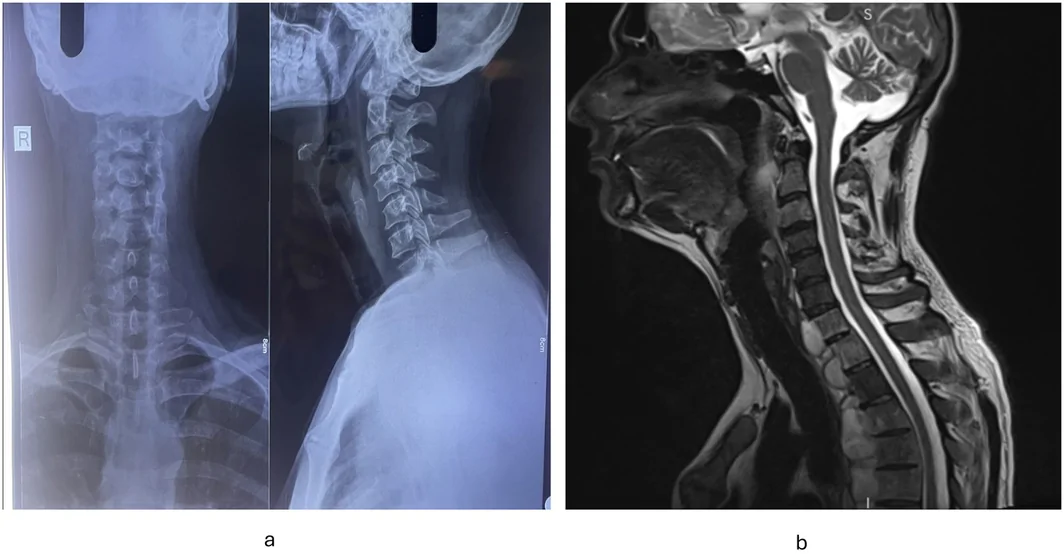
(a) Plain radiograph of the cervical spine (AP and lateral views) showing increased prevertebral soft tissue shadow on the lateral view, suggestive of retropharyngeal soft tissue swelling. (b) Sagittal T2‐weighted MRI of the cervical spine showing a hyperintense prevertebral collection consistent with retropharyngeal abscess, causing anterior displacement of the posterior pharyngeal wall.
